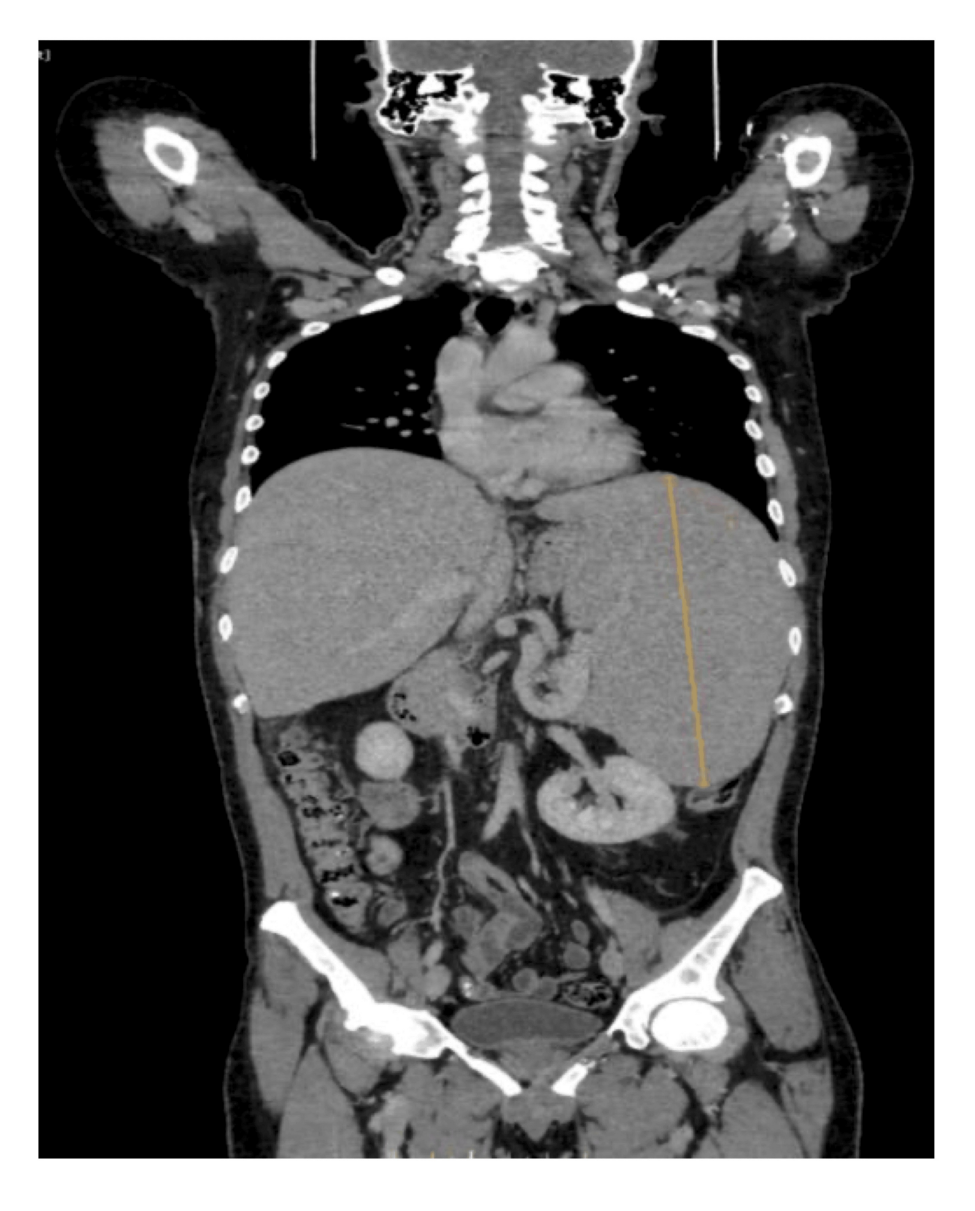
Contrast-enhanced abdominal CT (coronal view) performed at presentation, demonstrating massive splenomegaly measuring 24 cm craniocaudally; the liver graft appears homogeneous, without focal lesions or biliary dilation.
